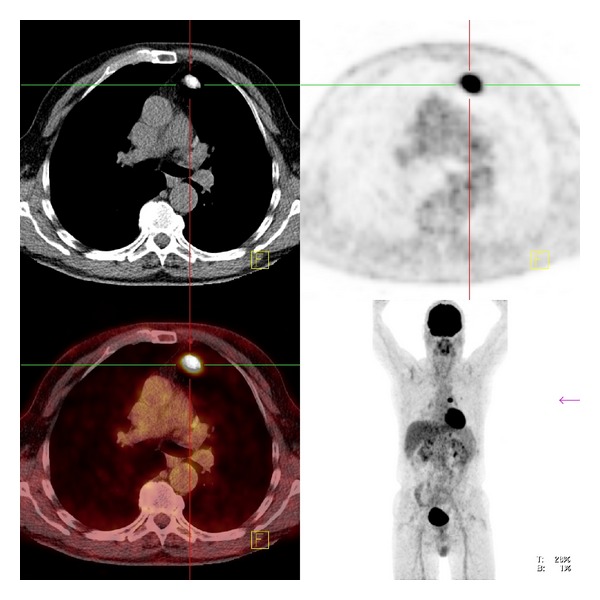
Pulmonary nodule with high FDG uptake ventrally in the left upper lobe.
